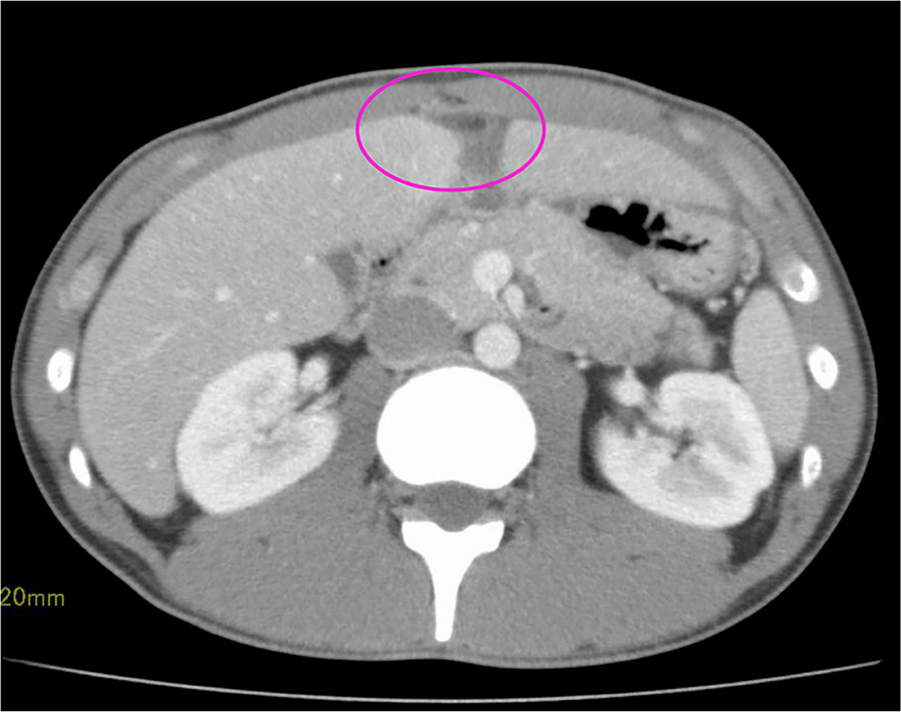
Contrast-enhanced computed tomography findings in Case 1. Thickening of the hepatic round ligament and increased surrounding fat concentration are seen (circle).
